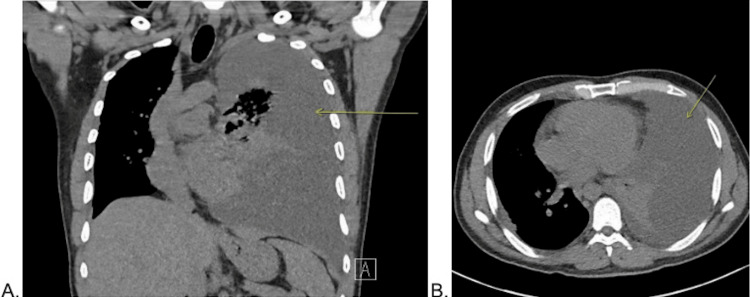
CT of the chest CT of the chest, coronal cross-section (A) and axial cross-section (B), indicating a large left-sided pleural effusion (arrow).
